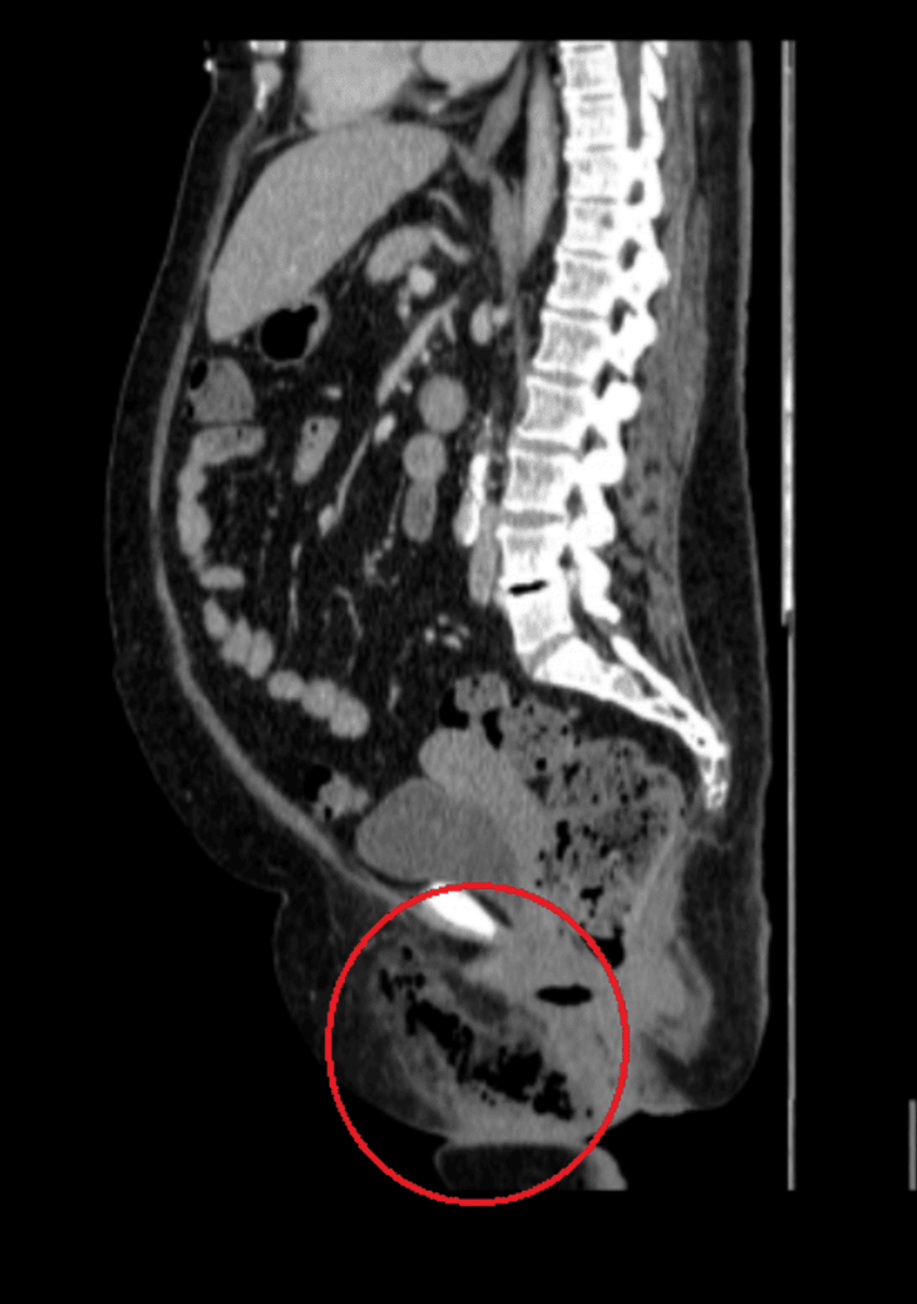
Gas in the subcutaneous tissues of the perineum in contrast-enhanced CT (sagittal plane) CT: computed tomography
